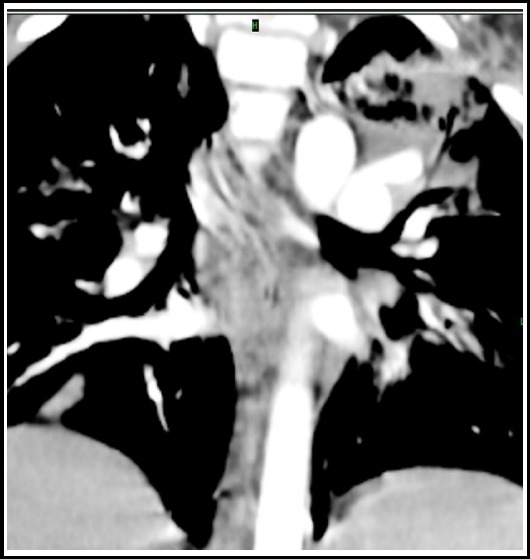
CT scan with IV contrast section in mediastinal window setting shows mediastinal fibrosis secondary to TB.
